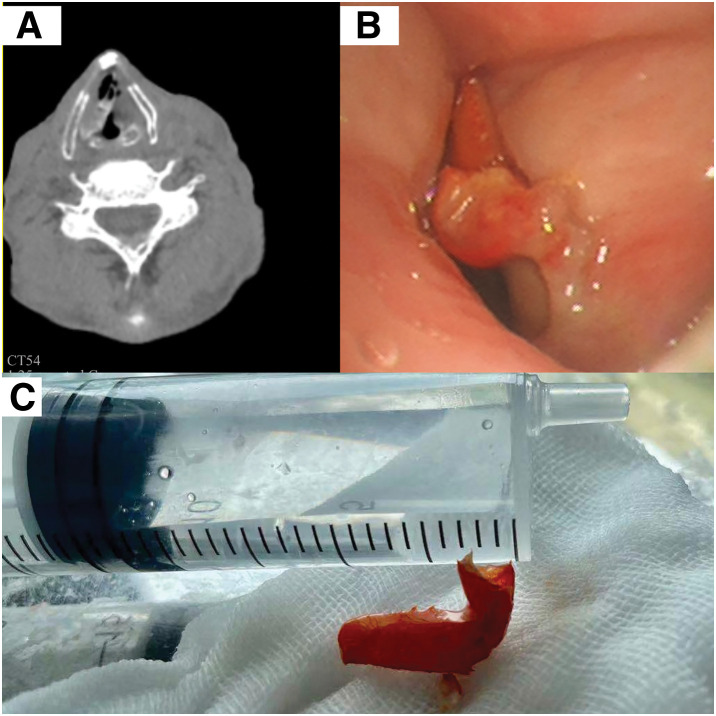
Crayfish claw lodged between the vocal cords, causing aphonia. (A) Cervical CT showing a tubular hyperdense lesion. (B) Flexible bronchoscopy demonstrating the claw between the vocal cords. (C) Retrieved crayfish claw.
